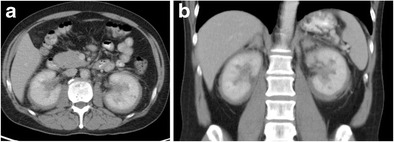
a A portal venous phase CT shows a soft tissue rind encasing both kidneys and extending into the renal sinus. b The coronal reconstruction shows underlying renal parenchyma appearing normal in this patient with Erdheim-Chester disease
